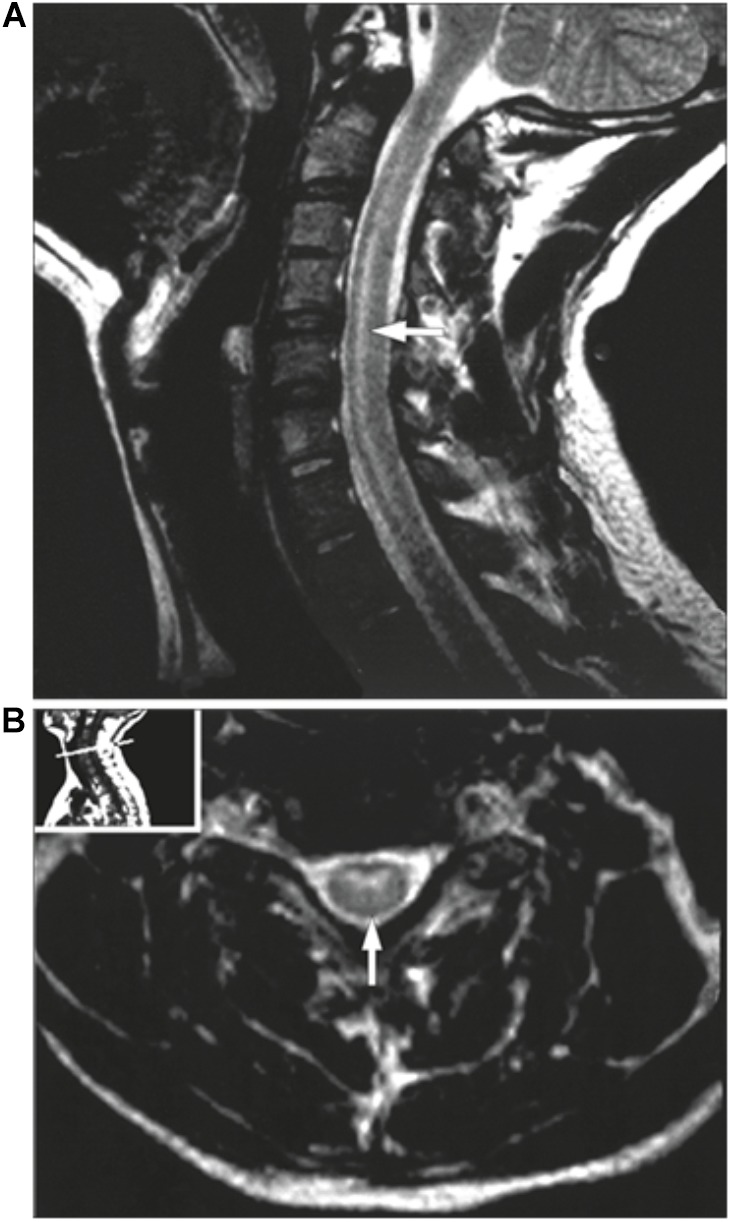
MRI of a poliovirus AFM patient. The MRI presents a sagittal (A) and an axial image (B) of the central nerves system. (A) presents a case showing hyperintensities involving the anterior horn cells from C3 to C7. (B) demonstrates the same case as an axial image. Taken from Haq and Wasay (2006). Order License Id: 4382500944212.
